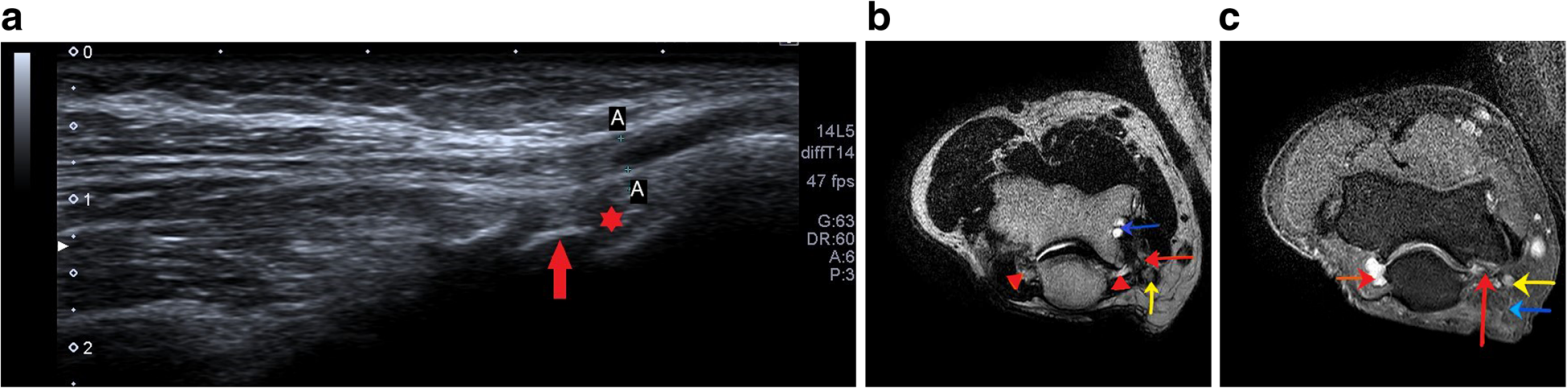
a Hypoechoic ulnar nerve (A) at the elbow with underlying synovial thickening (red star) and cortical irregularity (red arrow). b Thickened hyperintense ulnar nerve (yellow arrow) with surrounding synovial thickening (red arrowhead) and joint effusion on T2-weighted MR image. Also noted is subchondral cysts (blue arrow) and cortical irregularity (red arrow). c PD FS image shows hyperintense ulnar nerve (yellow arrow), joint effusion (red arrow) and panniculitis (blue arrow), all features of chronic inflammation
